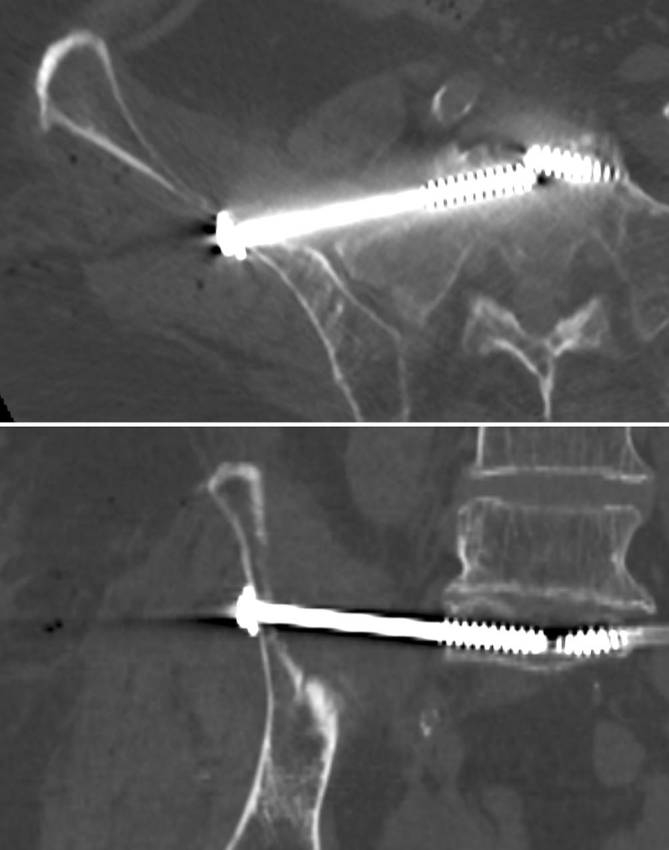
Screw misplacement—postoperative computed tomography (CT) control. Same patient with sacral dysmorphism as in Figs. 4 and 8. The patient showed a foot drop immediately after surgery. The CT control showed a partial extraosseous screw trajectory (“in-out-in”) with lesions of the L5 nerve root
